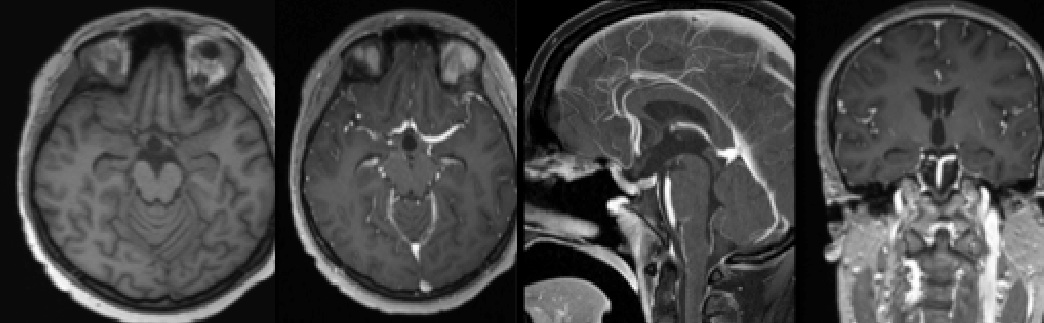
Six-month Postoperative Brain MRI
